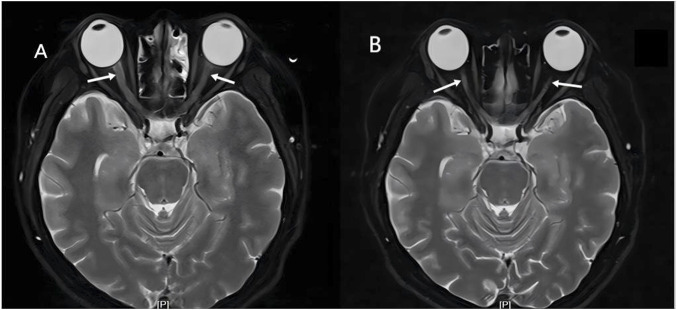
Day 9 post-exposure optic nerve MRI: bilateral optic nerve swelling with patchy T2WI hyperintense signals and marked contrast enhancement on contrast-enhanced imaging (A). Day 154 post-exposure optic nerve MRI: optic nerve without swelling, slightly thinned, showing patchy T2WI mildly hyperintense signals; moderate contrast enhancement with reduced intensity on contrast-enhanced imaging (B).
